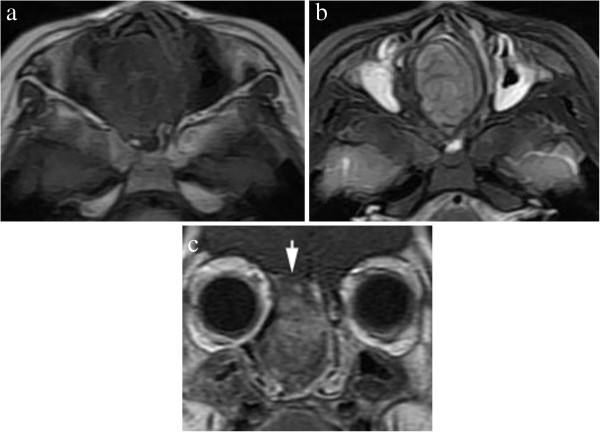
CT of sinonasal cavity. (a) An axial non-contrast CT revealed an ovoid soft-tissue mass in the right nasal cavity. (b
